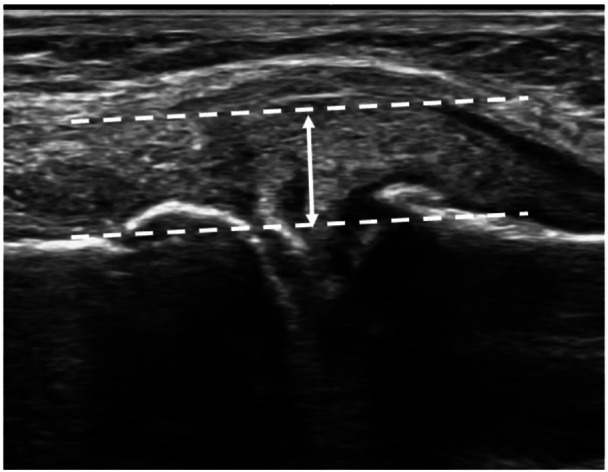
Ultrasonographic evaluation of medial meniscal extrusion. The baseline was drawn to connect the femur and the tibia without osteophytes. The target lines were drawn parallel from the baseline to the medial meniscus.
